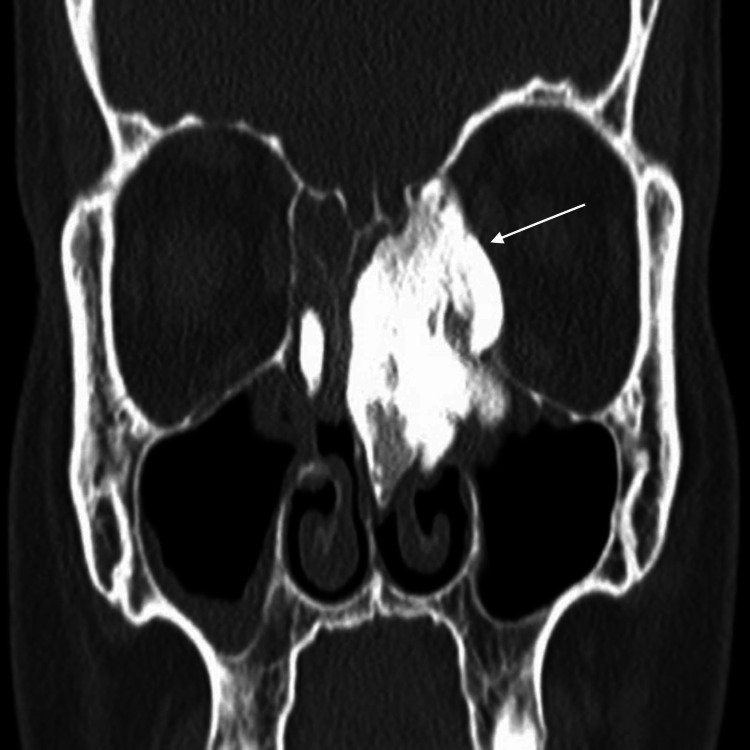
Preoperative CT scan: coronal view shows lesion extension from left ethmoid sinus into left orbital cavity.
